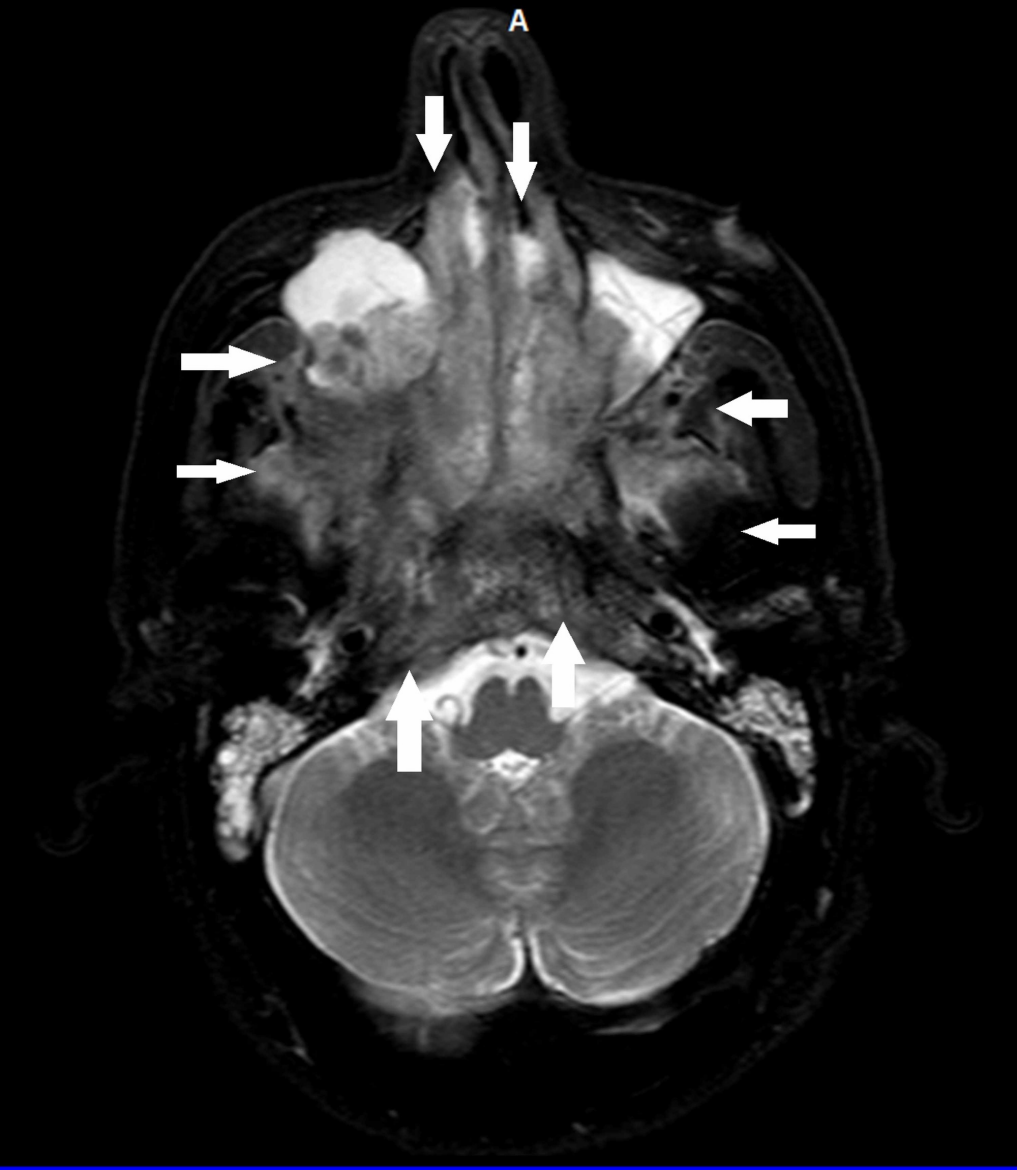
MRI scan depicting the size of the tumor extension (white arrows).
